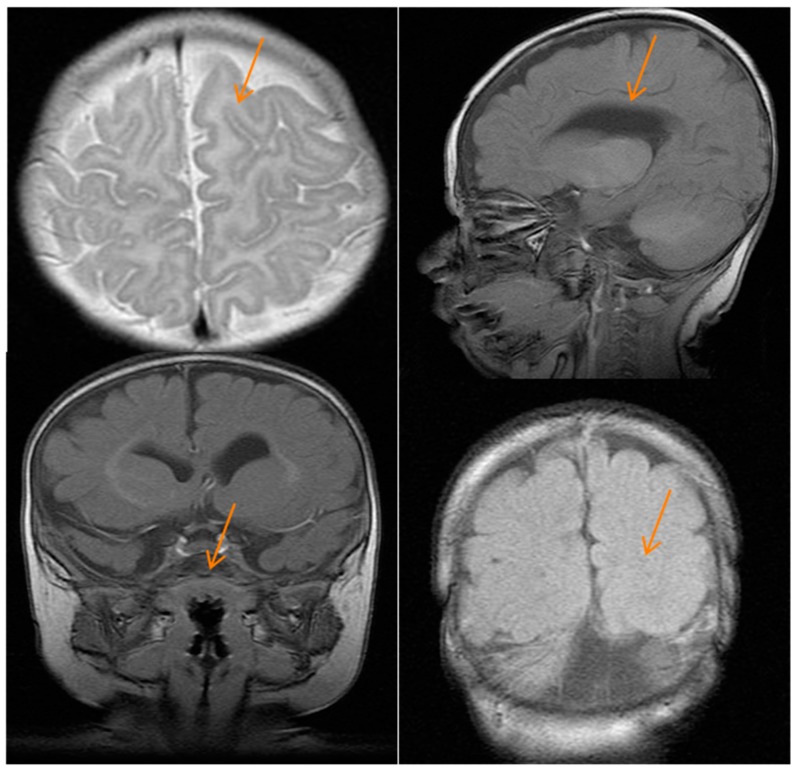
Brain magnetic resonance imaging of the patient. Images show cortical dysgenesis (top left and lower right figures), a thin corpus callosum (top right figure), and hypoplastic pons (lower left figure); the red arrows indicate the brain defects.
